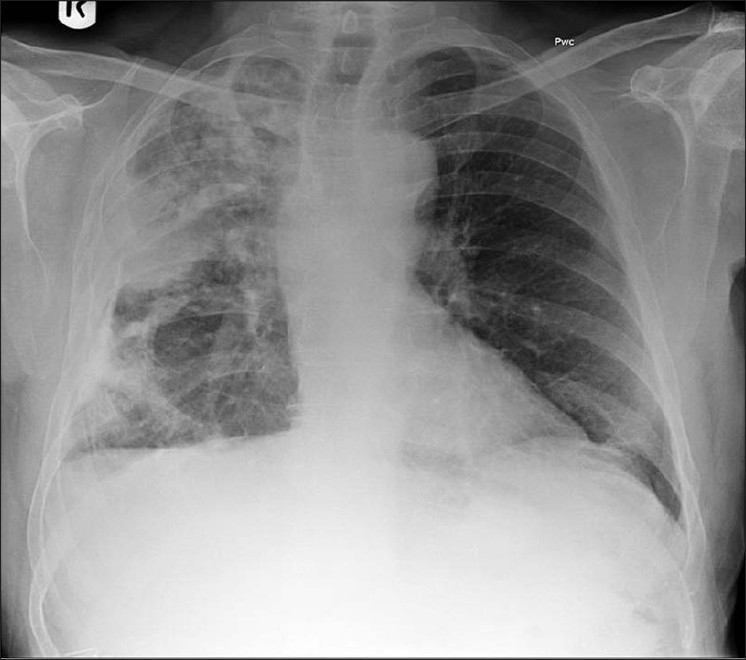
A chest radiograph of a patient with history of asbestos exposure shows multiple right-sided pleural plaques some calcified and a large pleural based PN raising the suspicion of a mesothelioma (See Figure 14)
